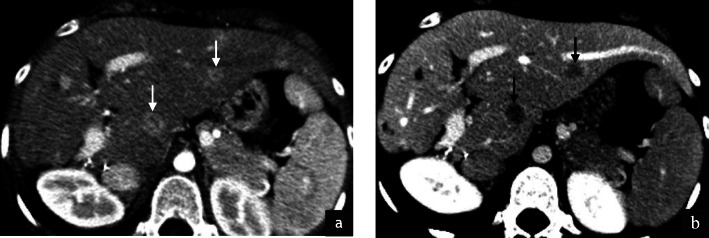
Hepatic metastases in a patient with alveolar soft part sarcoma. Biphasic CT (liver window) demonstrates marked arterial hyperenhancement of lesions in Segment III and V (a, white arrows). These demonstrate rapid washout in the portal venous phase (b, black arrows) in keeping with hypervascular metastases
